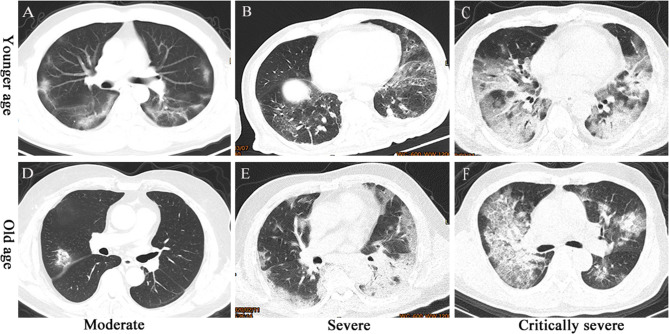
CT imaging of COVID-19 patients. (A) Moderate-severity pneumonia in a 36-year-old man presenting as ground-glass opacity under the pleura in both the lungs. (B) Severe pneumonia in a 50-year-old man presenting as ground-glass opacity and large exudative shadows in both the lungs. (C) Critically severe pneumonia in a 58-year-old man presenting as large patches of exudative shadows in both lungs. (D) Moderate-severity pneumonia in a 63-year-old woman presenting as ground-glass opacity in the right upper lobe. (E) Severe pneumonia in a 78-year-old woman presenting as large exudative shadows in both lungs. (F) Critically severe pneumonia in a 69-year-old man presenting as large patches of ground-glass opacity and exudative shadows in both lungs.
